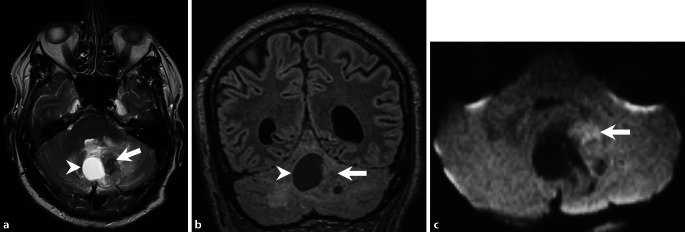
Axial T2 weighted images (a) and coronal fluid attenuated inversion recovery (FLAIR) images (b) confirmed a space-occupying intra-axial cerebellar lesion. The lesion comprised a sizable cystic portion (a + b, *arrowhead*) adjacent to a solid tumor component (a + b, *arrow*). Axial diffusion-weighted MR images (b-value: 1000) indicated restricted diffusion in parts of the solid tumor component (c, *arrow*)
